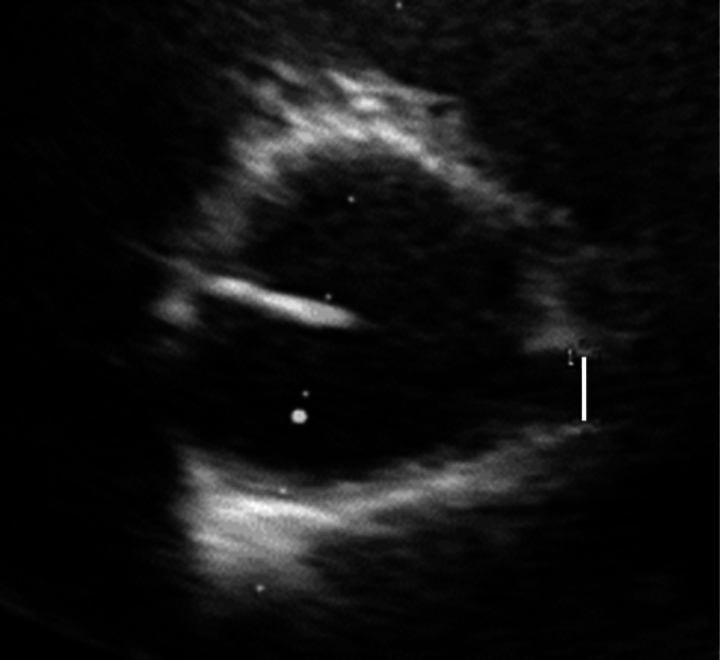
Transthoracic echocardiography. Parasternal cross-sectional view shows the dilated origin (white line) of the right coronary artery.
